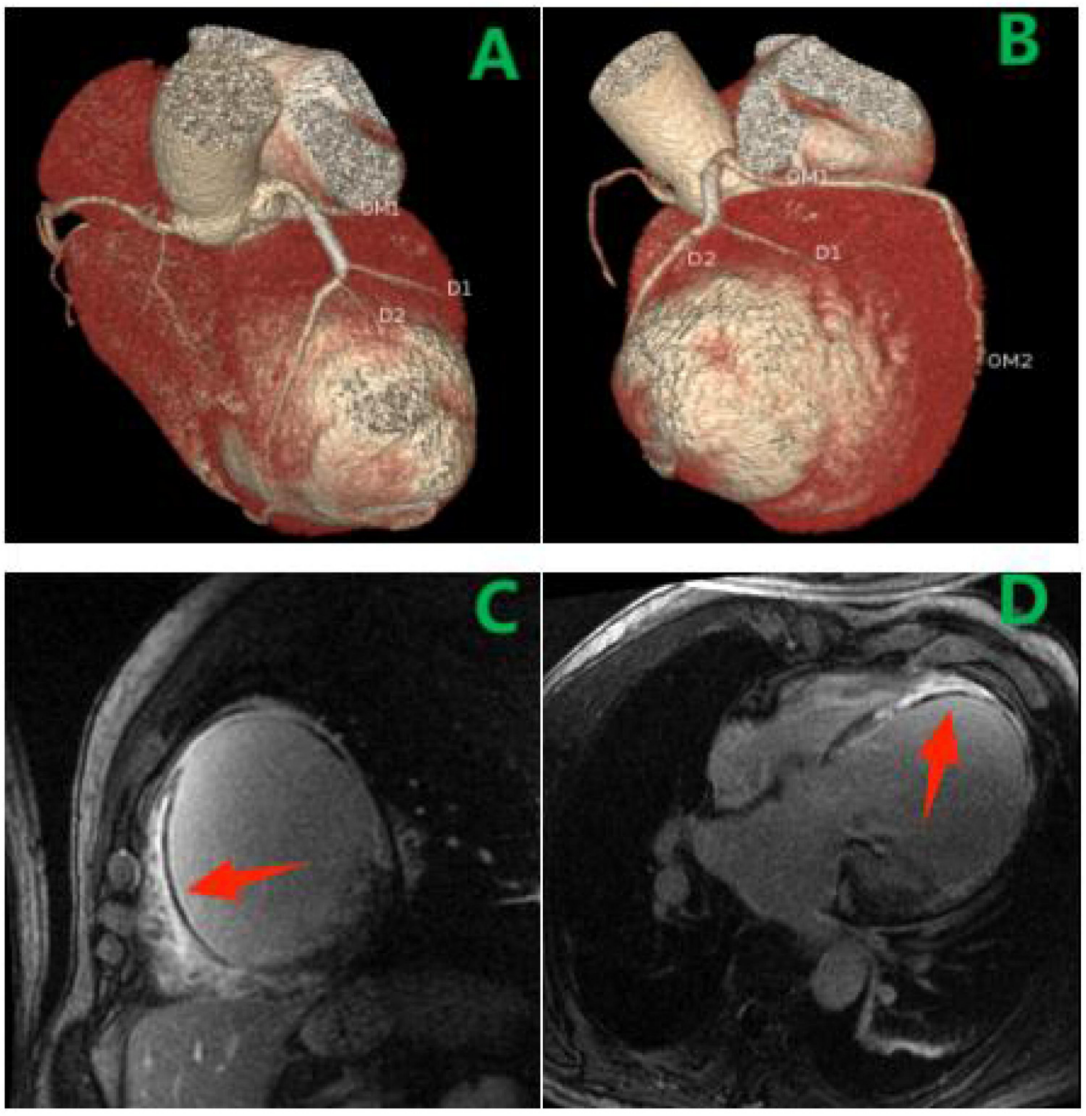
(A,B) CTA: giant aneurysm adjacent to LAD. (C,D) CMR LGE confirmed microvascular obstruction (MVO) within the apical septal subendocardial infarct (arrow).
